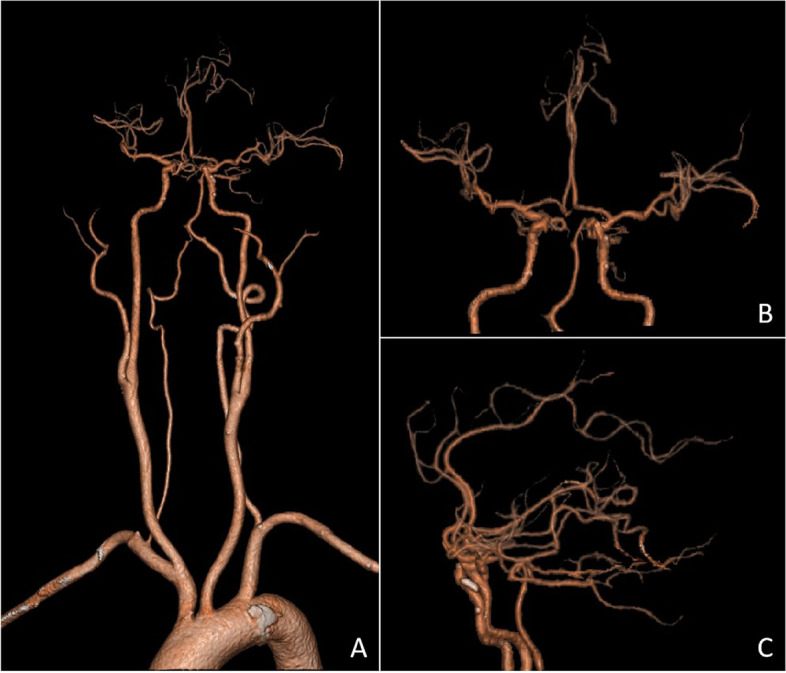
CTA of the head and neck was repeated 12 h after surgery. A. suggested a plaque in the left internal carotid artery, but no significant stenosis. B and C the middle, anterior and posterior cerebral arteries were not occluded as shown in frontal and lateral CTA of the head
